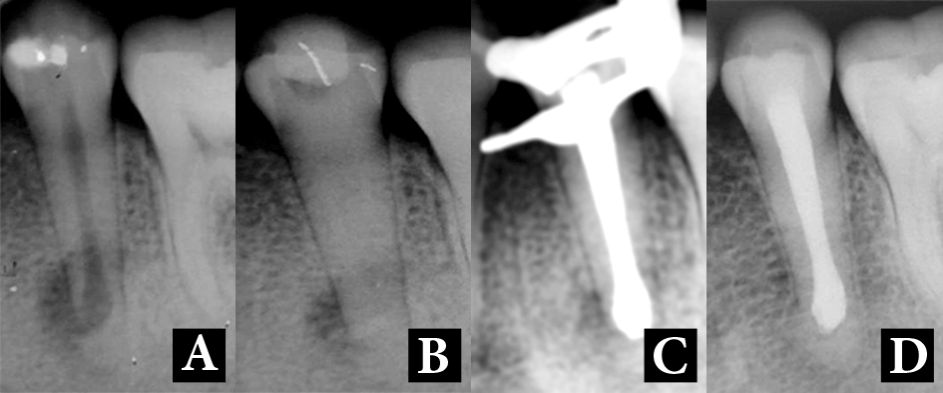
A*) Preoperative radiography of the mandibular left second premolar; *B*) Root canal dressing with calcium hydroxide paste; *C*) Canal obturation after induction of apical barrier; *D*) Six-year follow-up
